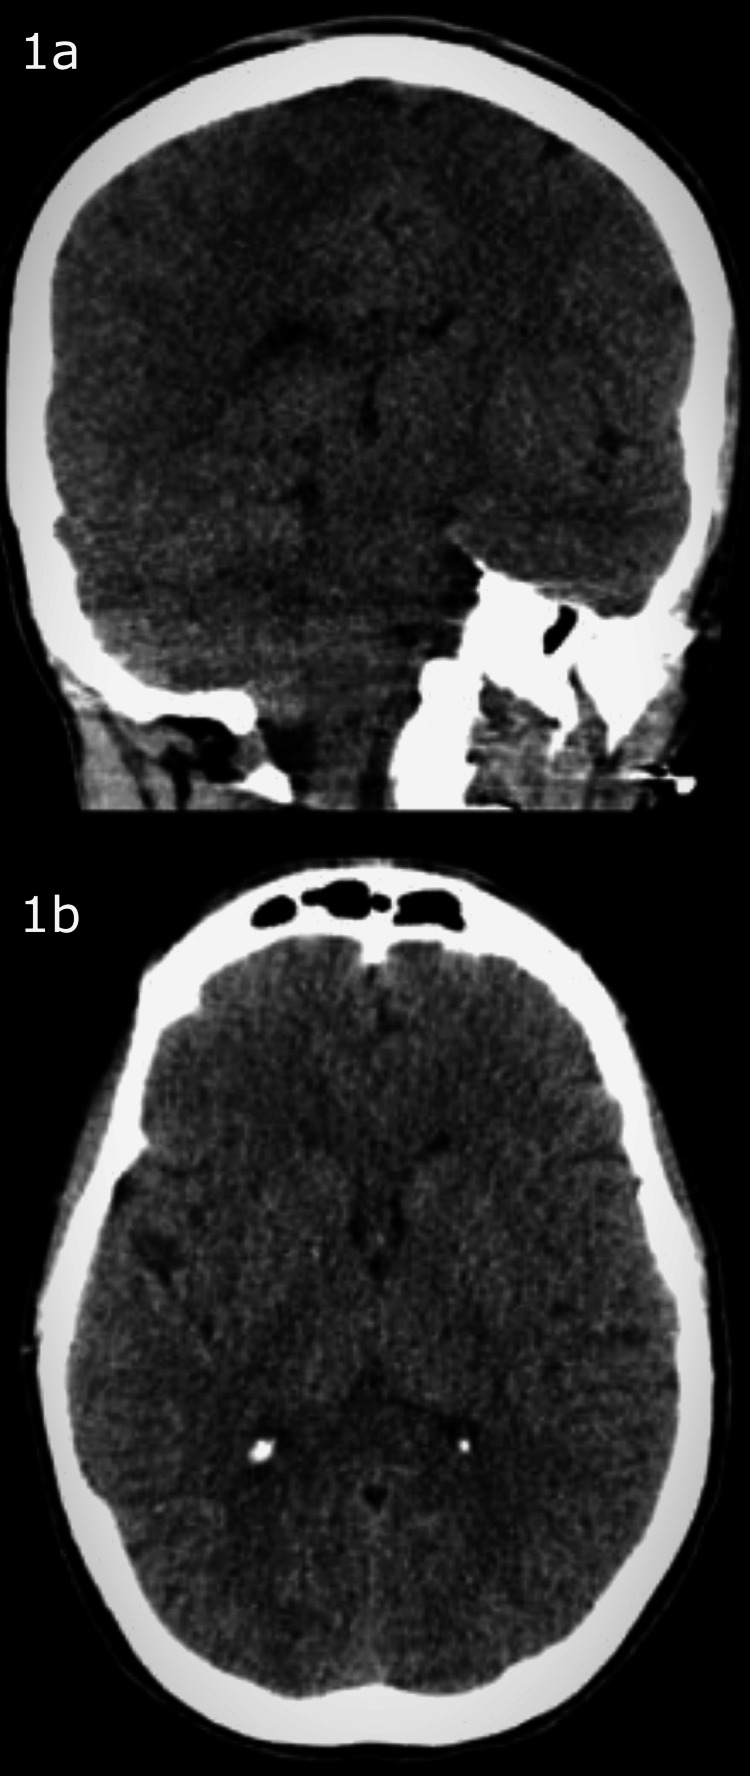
CT brain without contrast 1a: coronal view without contrast showing normal integrity of bony, soft tissue, and vascular structures. 1b: horizontal view without contrast showing normal integrity of bony, soft tissue, and vascular structures CT: computed tomography
